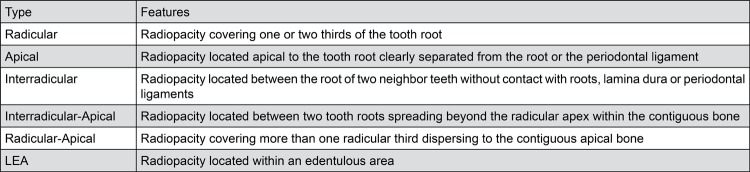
Radiographic types of GOLs
